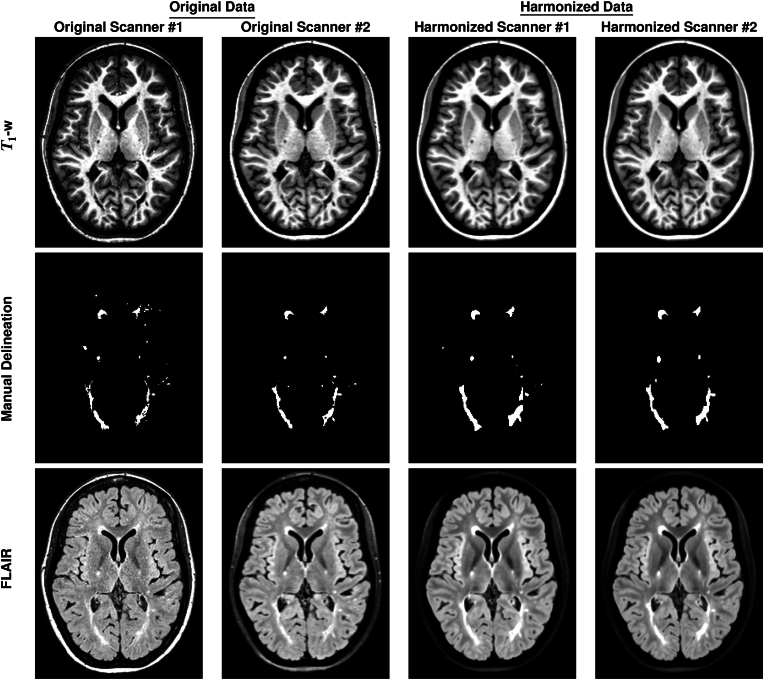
Shown are example images for one subject on both scanners, original and harmonized; as well as the corresponding manual masks. From left to right the columns are: Original Scanner #1, Original Scanner #2, Harmonized Scanner #1, and Harmonized Scanner #2. From top to bottom the rows are: *T*_1_ − *w*, Manual Delineation, and FLAIR. The *T*_1_ − *w* images are either MPRAGE or MEMPRAGE, see Table 2 and Sec. 3.2 for the complete details. The SDI for the original data for this subject is 0.572 and for the harmonized data is 0.795. For a different subject, we show a magnified portion of the FLAIR images and corresponding delineations in Fig. 4.
